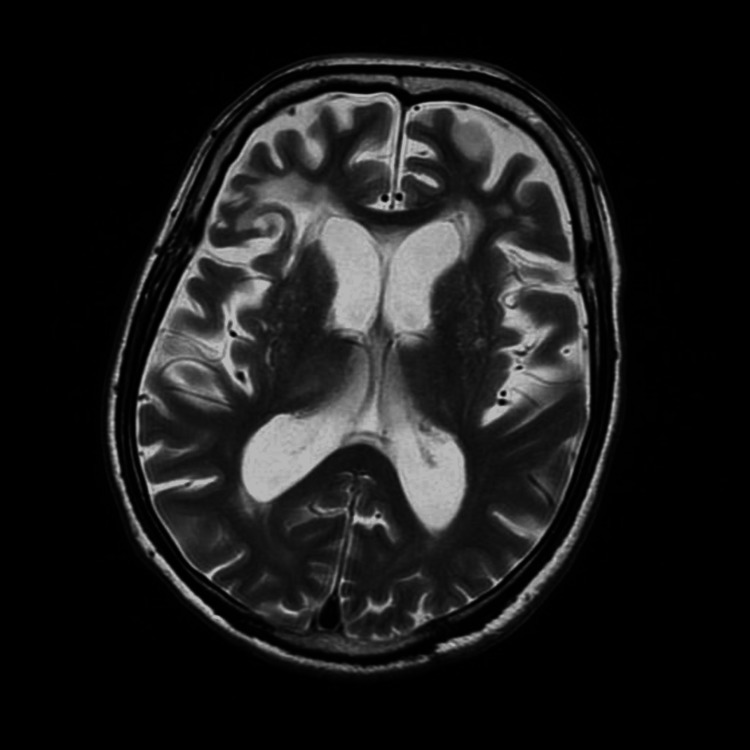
T2-weighted image shows cerebral infarctions of the bilateral cerebral hemispheres
